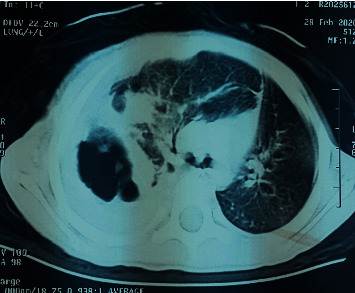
CT scan showing overdistension of the right chest with parenchymal air cystic lesions involving the external segment of the middle lobe.
